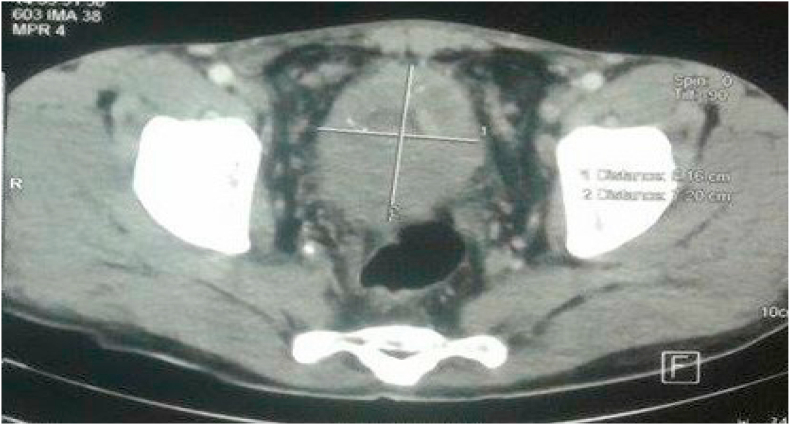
Abdomino-pelvic CT scan demonstrated a large perivesical process with endo and exo-vesical development related to the urachus tumor.
